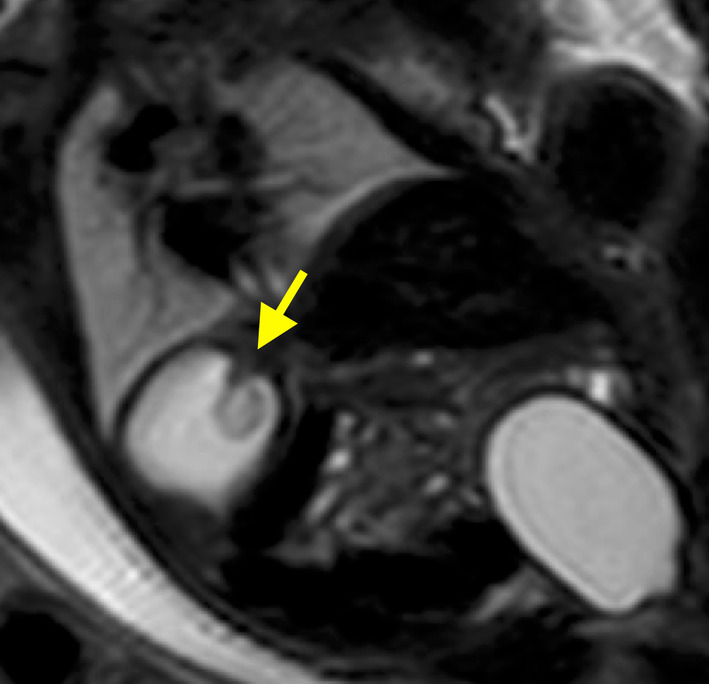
Coronal view of a T2-weighted image from a magnetic resonance imaging study. Obtained at 35 weeks of gestation. A low-intensity polypoid lesion measuring 11 mm in diameter is observed at the gastric fornix (arrow)
